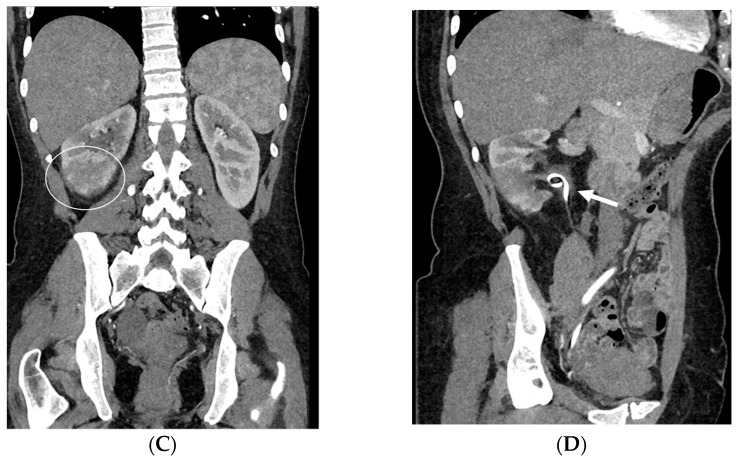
Acute pyelonephritis after stenting procedure. CT portal-phase images in the axial (A,B), coronal (C), and curved MPR (D) views demonstrate multifocal regions of diminished enhancement in the medio-inferior region of the right kidney when compared to normal portions of the kidney and a contralateral one (circle). The swirl of the stent is evident in the pelvis (arrow).
